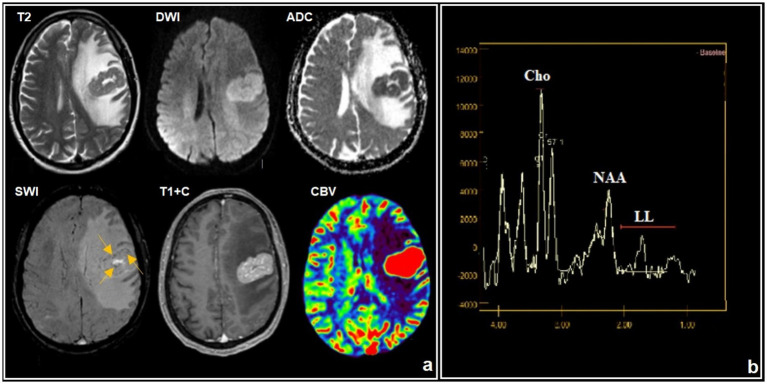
A 39-year-old HIV-positive male, with atypical PCNS-LBCL with right-sided weakness and disturbance in consciousness for 1 month. The CD4^+^ lymphocyte count was 180 cells/μL: (a) conventional MRI, (b)
